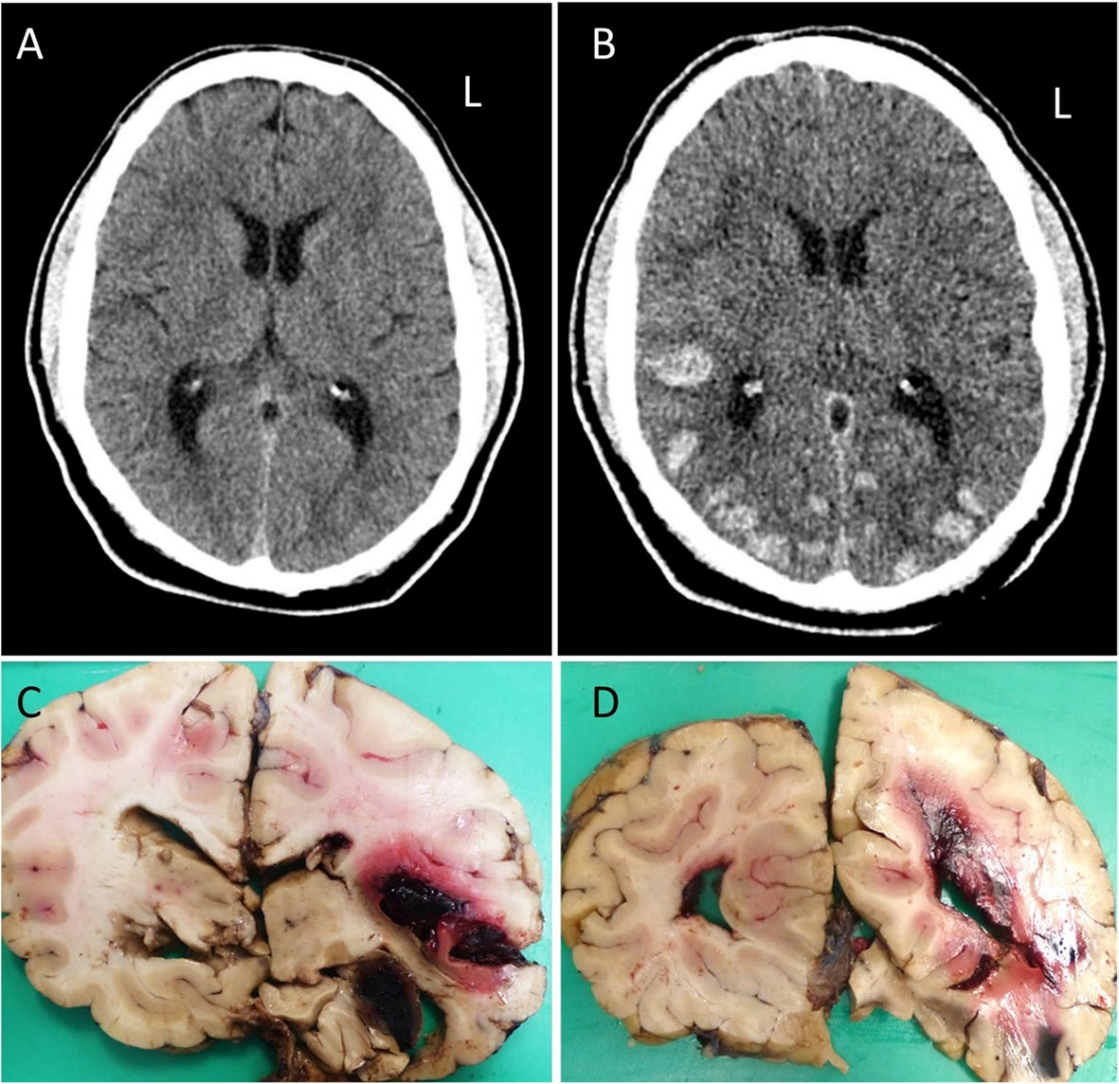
a Pre-thrombolysis non-contrast brain CT head scan demonstrating normal appearances, and in particular no evidence of haemorrhage. b After thrombolysis multiple foci of intracerebral haemorrhage with bilateral posterior hemispheric predominance are evident. c-d Macroscopic images of coronal brain sections showing multiple haemorrhages. There are recent intraparenchymal haemorrhages at the right insula and superior temporal gyrus (c) and the right occipital lobe (d). Extension to the subarachnoid space and the inferior (c) and posterior horns (d) is evident. Mild midline shift to the left is also present and the right uncus is partly necrotic (c)
